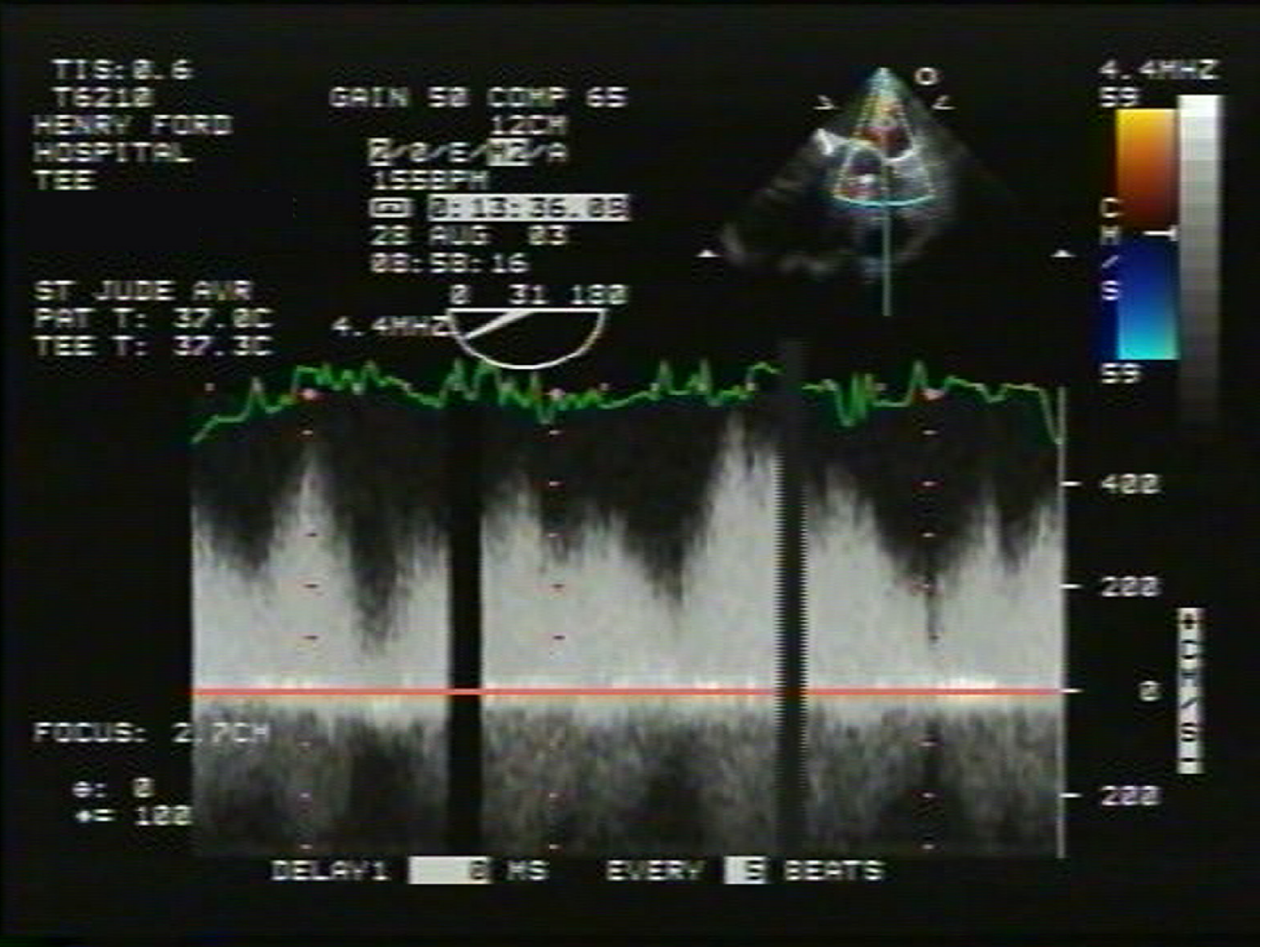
Continuous wave Doppler signal across turbulent jet showing high velocity throughout cardiac cycle but predominantly in systole consistent with aorto-left atrial fistula
